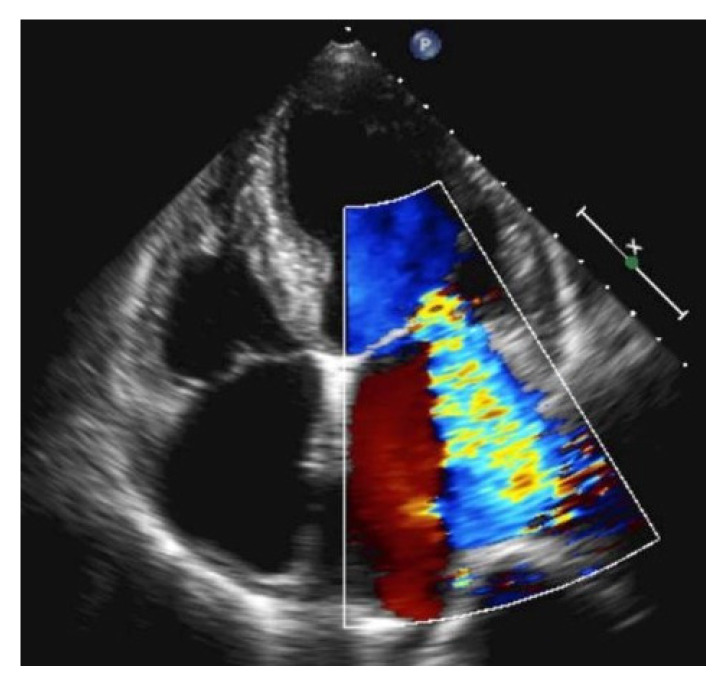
Large mitral regurgitation in apical four chamber view reaches the apex of the left atrium.
